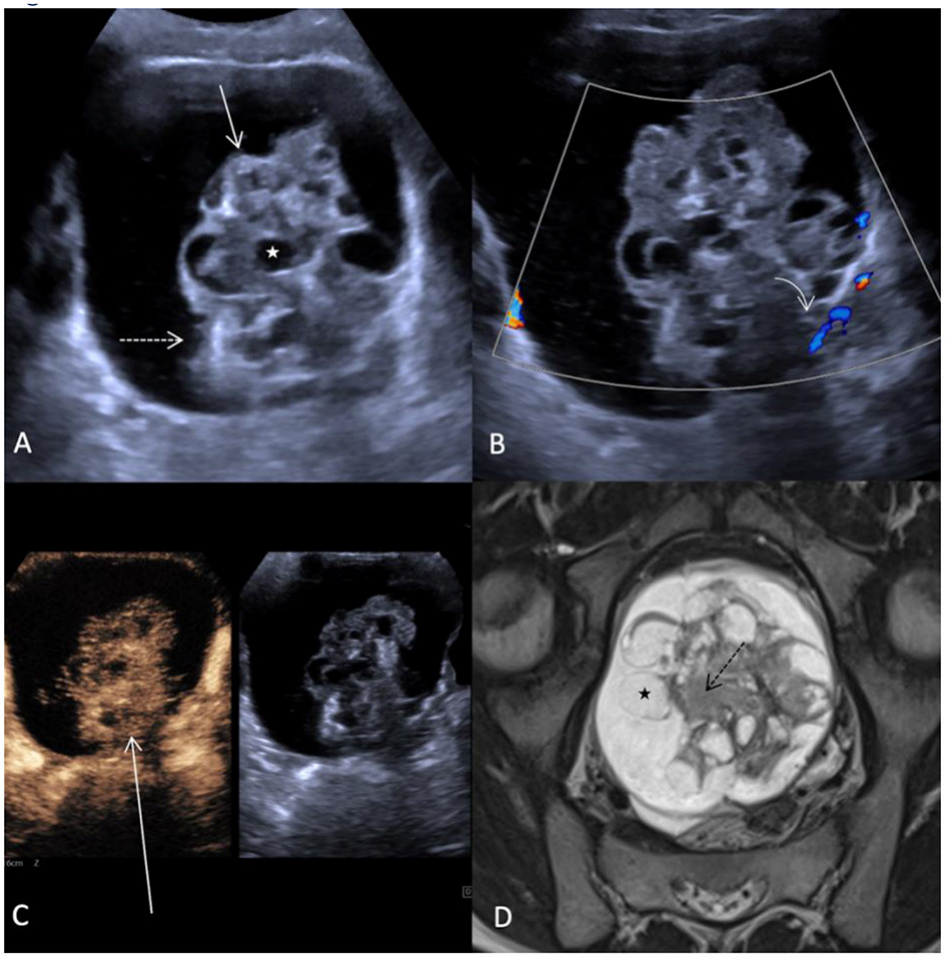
Case 2, 10-year-old girl with an immature teratoma with potential malignant changes. (A) B-mode ultrasound showing a cystic structure extending from the left ovary up to 16 cm in diameter. Within the cyst there is a solid part (arrow) measuring up to 7.5 cm. This is interspersed with an irregular surface (dashed arrow), inhomogeneous structure with multiple small cystoid lesions (white star). (B) In Doppler ultrasonography, no increased perfusion can be detected in the solid part (curved array). (C) Distribution pattern of contrast bubbles within the solid parts after 2 min. Strong enhancement with bizarre distribution within the irregularly configured solid mass (long arrow). (D) MRI of the pelvis shows the bizarre aspect of the solid mass with hyperintense cysts (black star) and irregularly configured hypointense stroma (black dashed arrow), (T2 TSE in paracoronal orientation).
